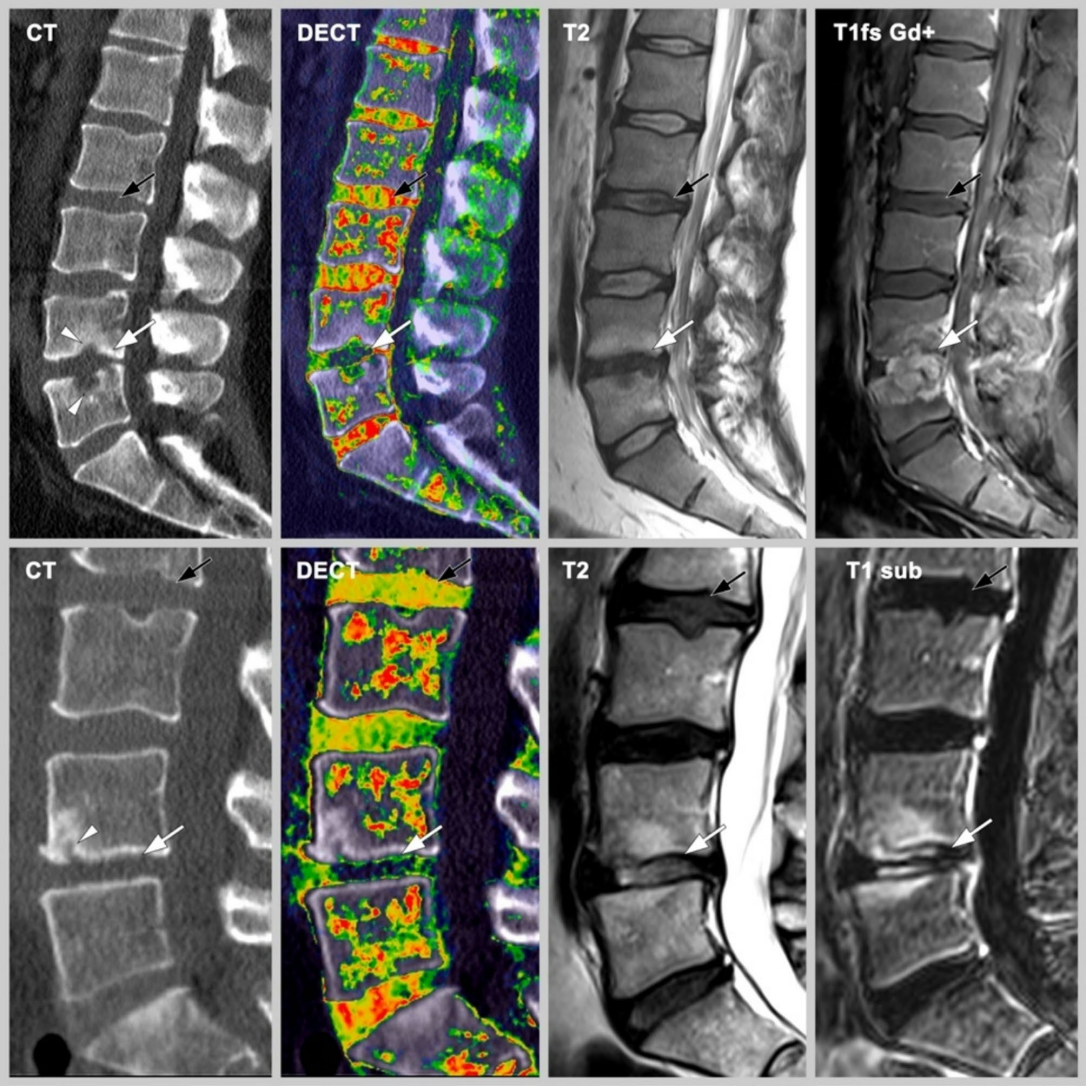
Images obtained in two patients with different spinal abnormalities. Images are sagittal reformations at 3 mm slice thickness and include conventional CT images, DECT cMaps, and T2-weighted MR images, and T1-weighted MR images T1fs Gd + and T1 sub. Patient 1 (upper row): Spondylodiscitis of the L4/5 disc (white arrow). Patient 2 (lower row): Disc degeneration (white arrow) with erosion (white arrowhead) at the L4/5 level. The black arrows point to normal discs for comparison, respectively.*CT: Computed tomography; cMaps*: Collagen-/chondroitin-sensitive map; *DECT: Dual-energy computed tomography; MRI: Magnetic resonance imaging*.
